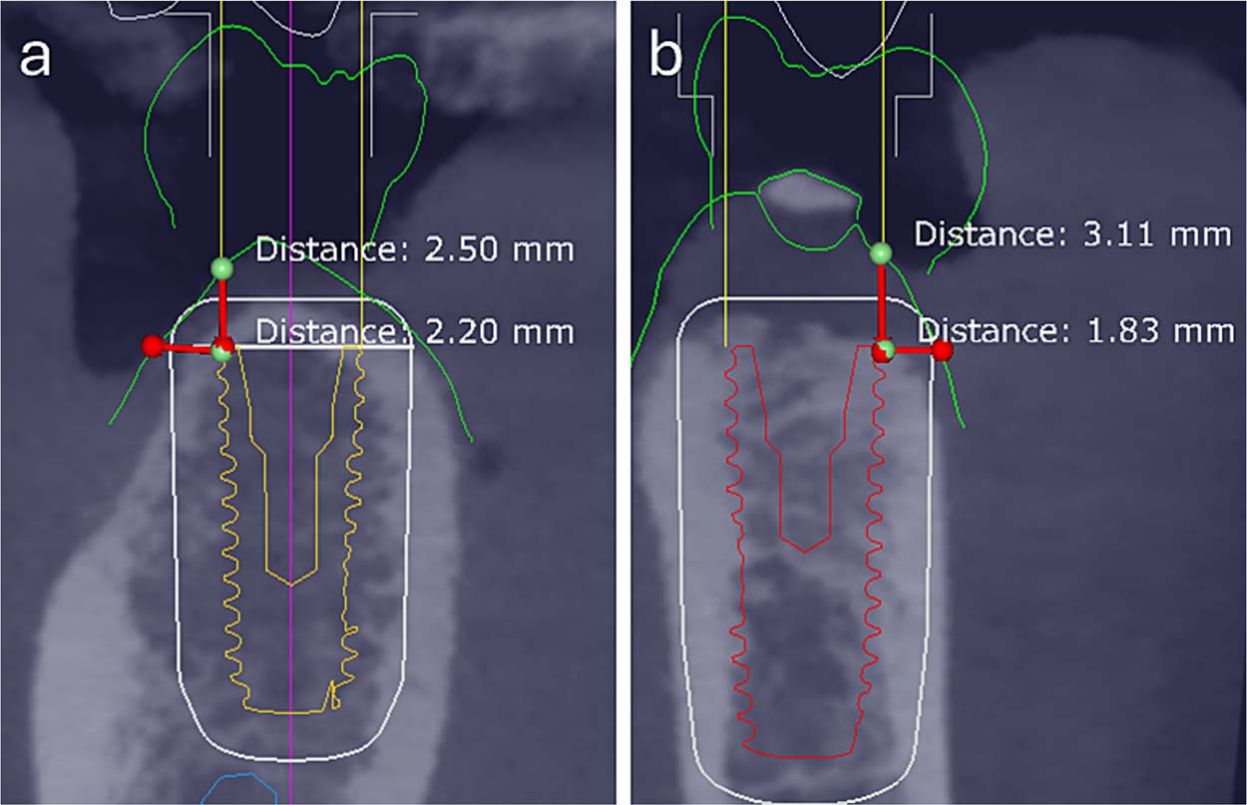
Preoperative digital assessment of buccal soft and hard tissue thickness using cross-sectional CBCT planning views. The measured distances represent the combined buccal bone and mucosal thickness from the buccal crest of the planned implant to the external surface of the mucosa. (a) Case 1: Total mid-buccal tissue thickness ≈ 2.20 mm; vertical thickness ≈ 2.50 mm. (b) Case 2: Total mid-buccal tissue thickness ≈ 1.83 mm; vertical thickness ≈ 3.11 mm.
